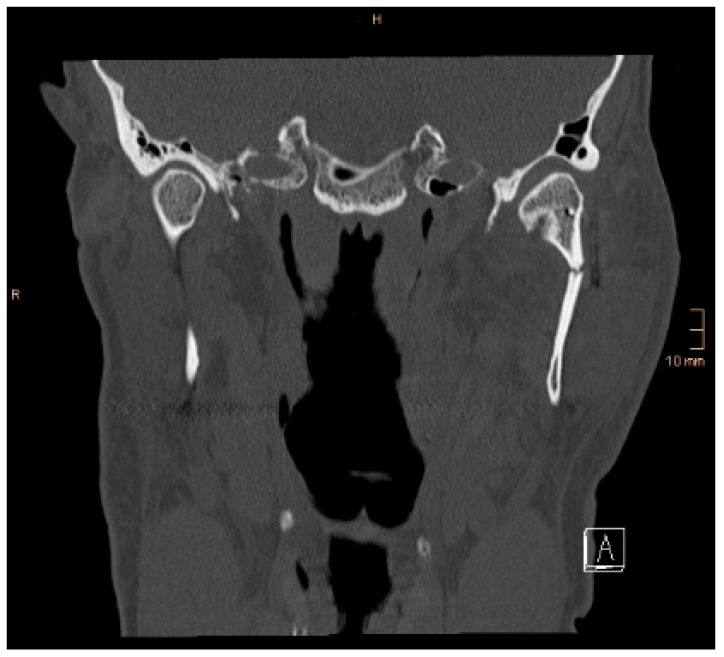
Coronal CT scan showing a condylar neck angulation on the left side after ORIF. An anatomical reduction could not be achieved.
